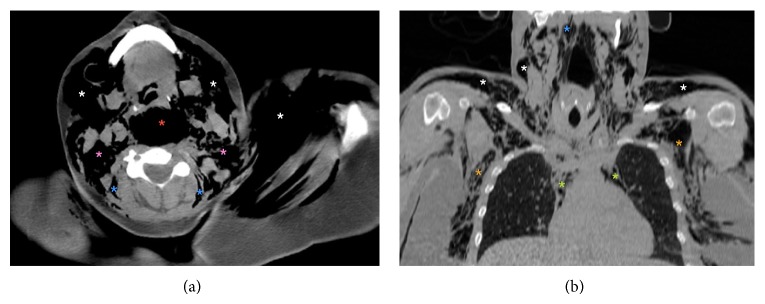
(a) Axial, (b)coronal cervicothoraco CT scan with 2D reconstruction obtained urgently.
